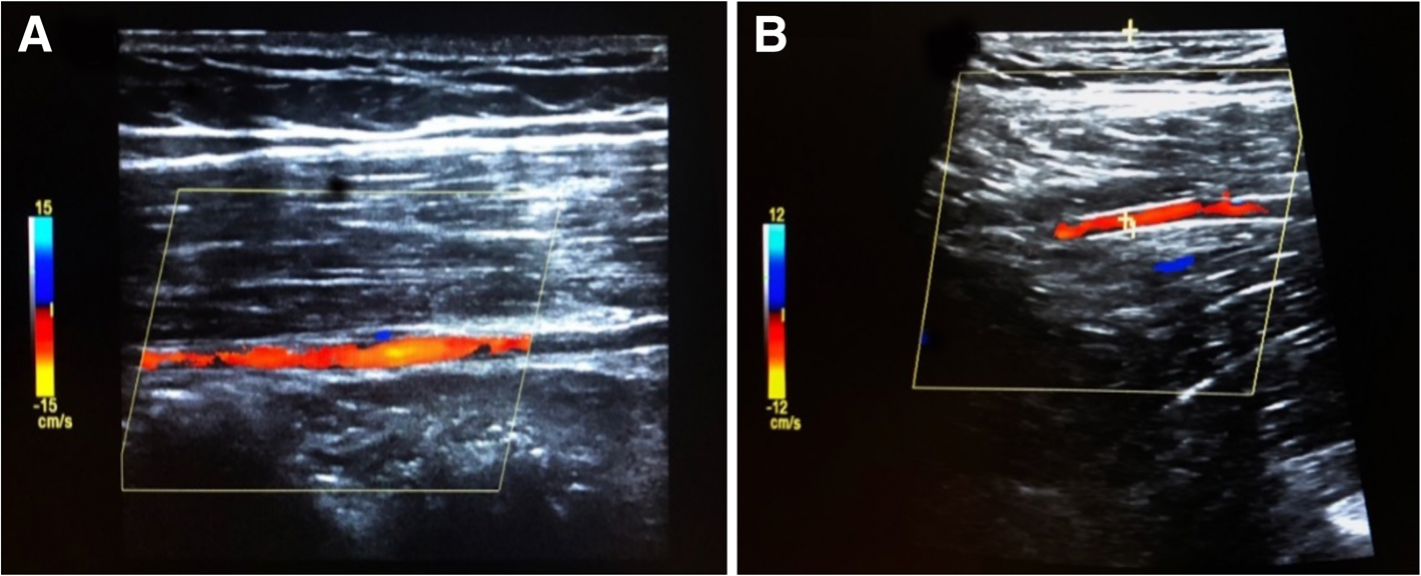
Mapping the a right inferior epigastric artery (IEA) and b deep circumflex iliac artery (DCIA) in a healthy volunteer using Doppler ultrasound
